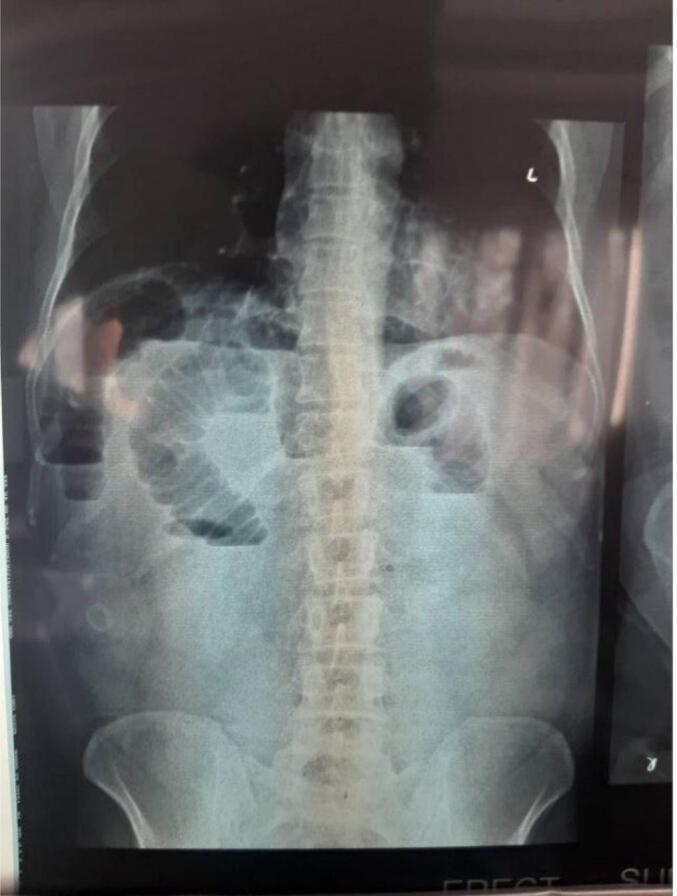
Plain X-ray Abdomen in Erect view showing air-fluid levels and a radio-opaque shadow in the right iliac fossa.
